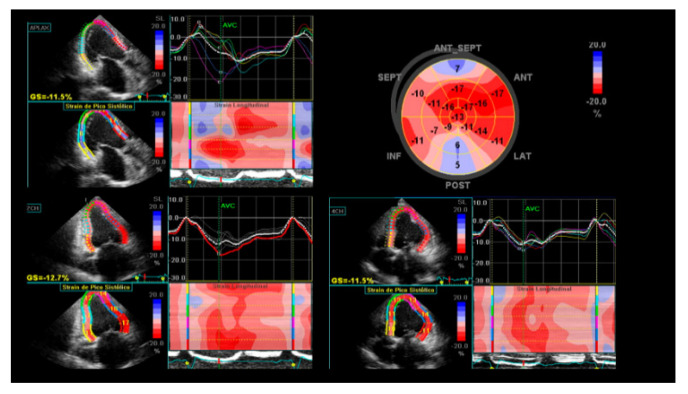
Global longitudinal strain of the left ventricle and strain curves depicting increased mechanical dispersion. Abnormal findings of left ventricular (LV) longitudinal strain in apical three-chamber view (top left); apical two-chamber view (bottom left); apical four-chamber view (bottom right); “Bull’s-eye” plot of strain values for each myocardial segment evidences anteroseptal and inferolateral akinesia represented by blue areas in the polar map (top right).
